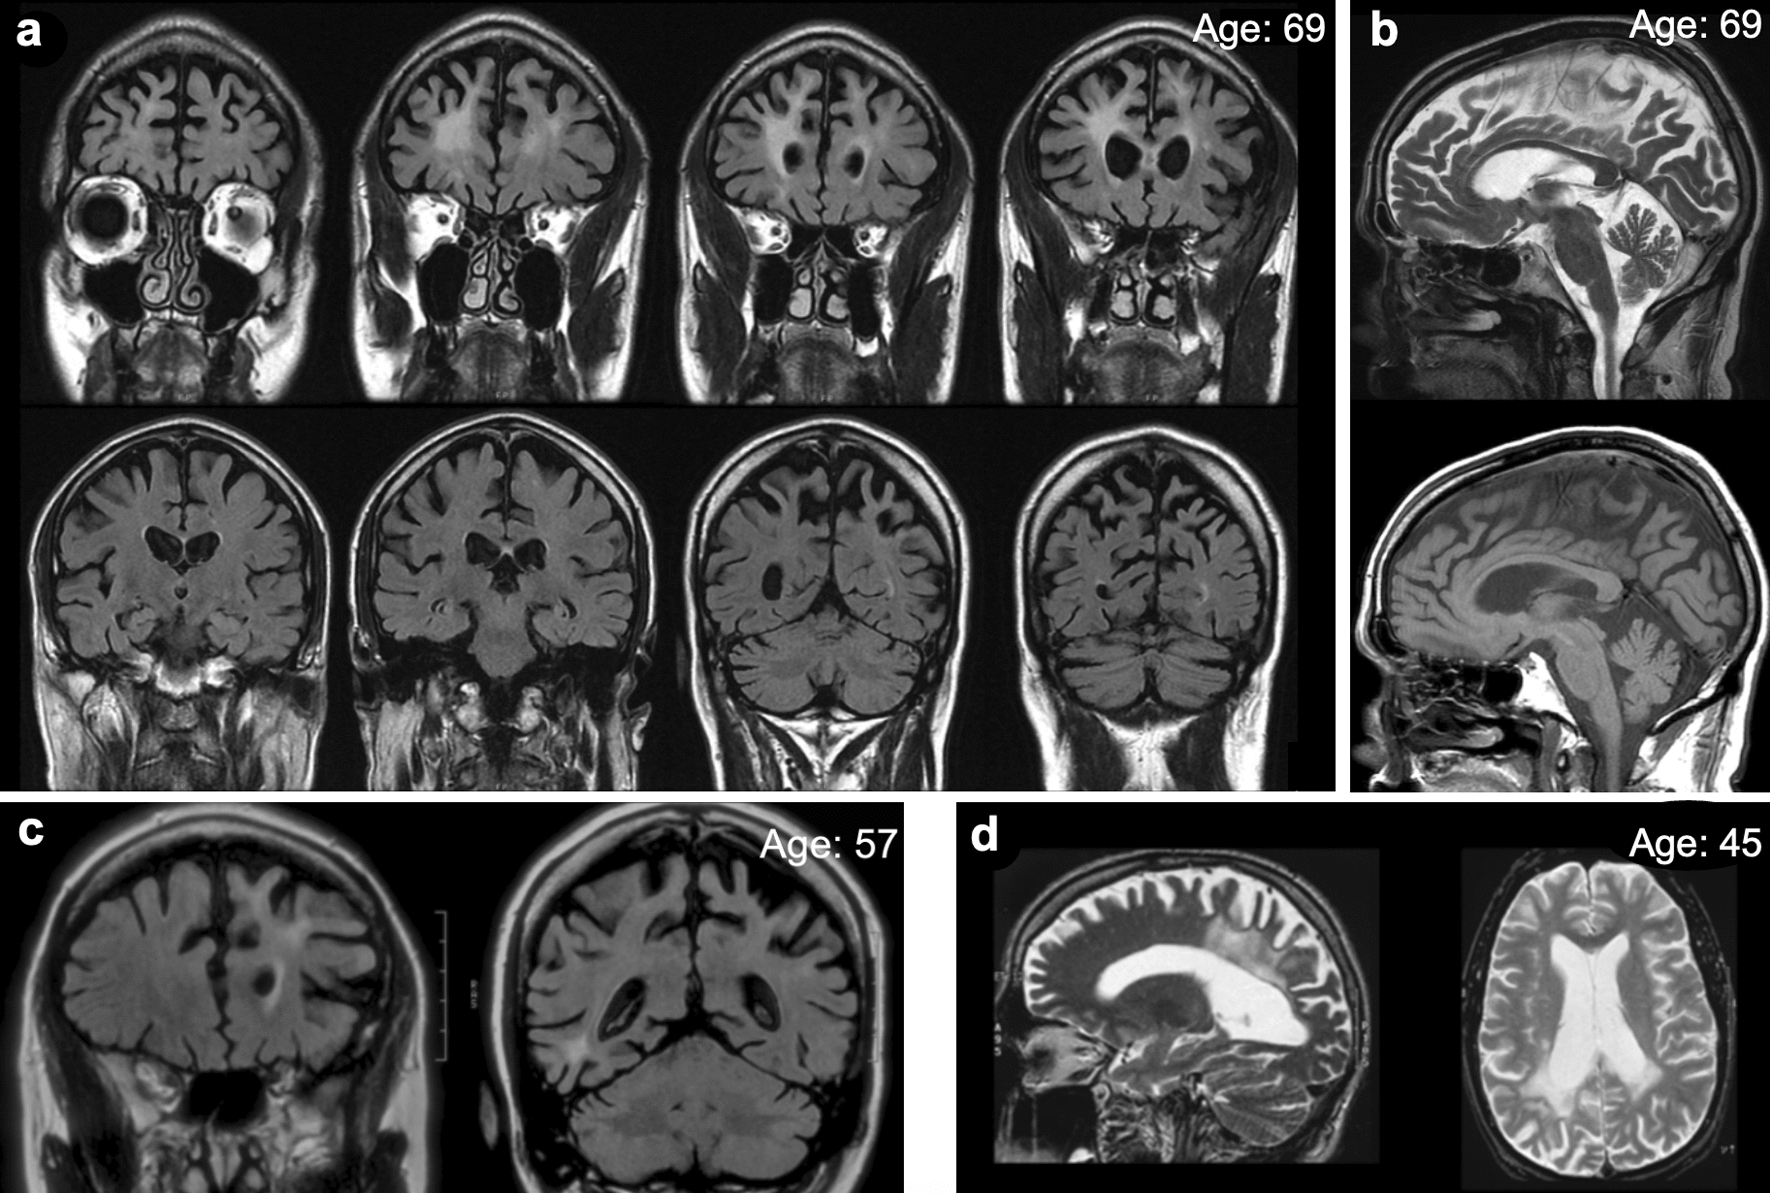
Proband’s MRI brain coronal FLAIR sequence a showed bilateral (right greater than left) hyperintensities in the subcortical white matter of the frontal lobes (upper row) associated with prominent bifrontal atrophy and (lower row) prominent biparietal atrophy with less impressive white matter changes. b Proband’s mid-sagittal T2-weighted image (top) and T1-weighted image (bottom) showed mild atrophy of the anterior aspect of the corpus callosum. c Affected sister’s coronal FLAIR sequence showed left greater than right frontal atrophy with mild white matter changes and prominent biparietal atrophy with focal white matter changes in the right temporal-occipital region. d Affected brother’s T2-weighted images showed prominent white matter hyperintensity in the posterior cingulate cortex (left side, sagittal view) and in the right greater than left lateral parietal cortex (right side, axial view). Atrophy was most prominent in the parietal lobes, with greater involvement on the right than the left
